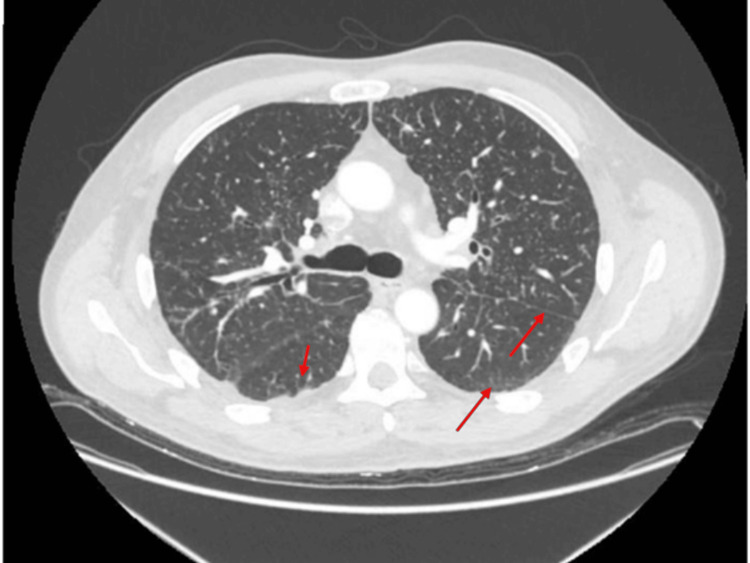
CT thorax (lung windows) demonstrating diffuse micro-nodules, interstitial thickening, and fissural beading. Arrows indicate the described abnormalities.
